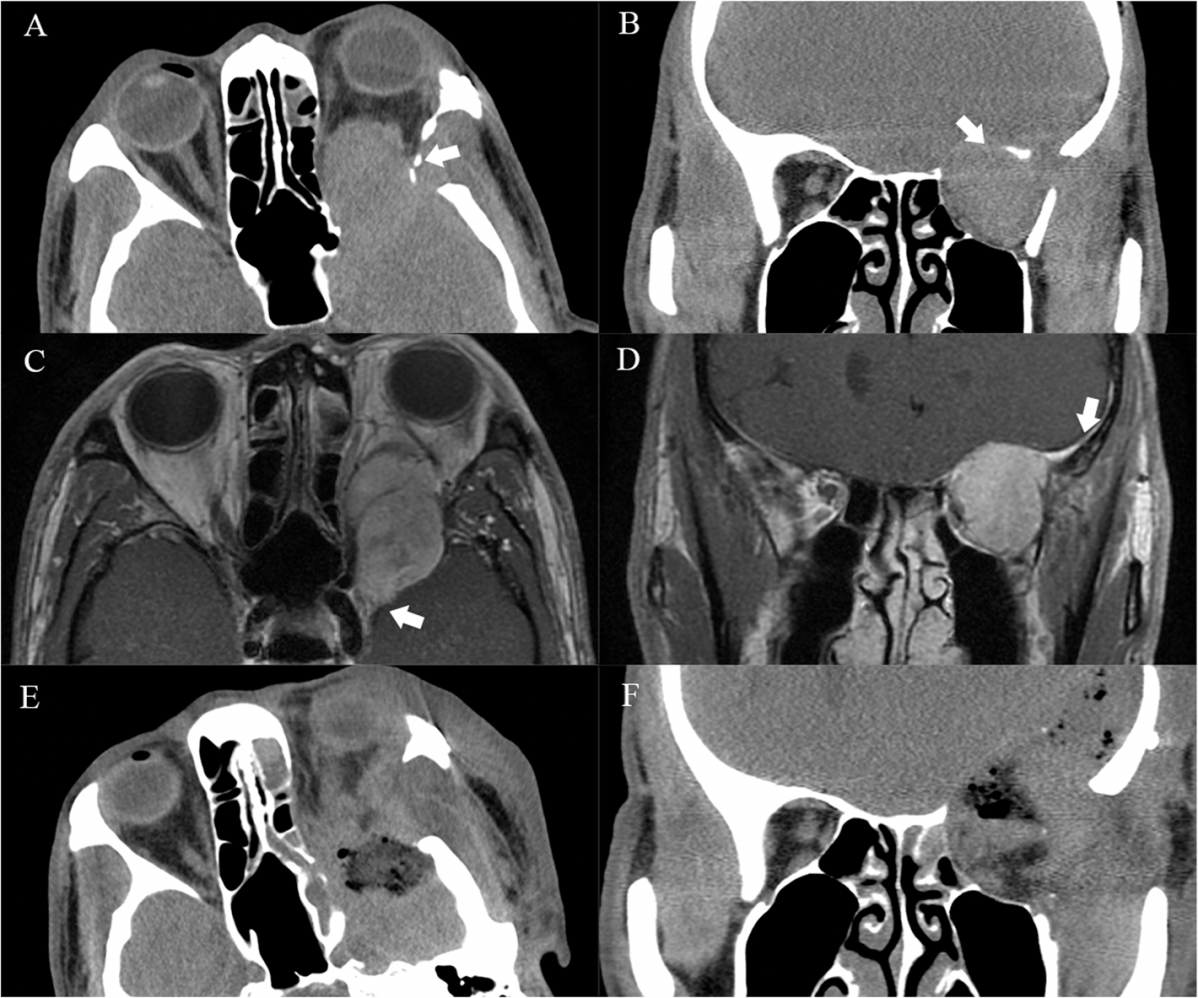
Case 6. The patient was a 48-year-old with a chronic disease process and left exophthalmos for more than 10 months. The patient underwent an operation (Transfronto-orbital approach) 6 years ago. a, b: CT showed that the tumor involved the bone of supraorbital bone with bone destruction (arrow). c, d: MRI showed that the main body of the tumor invaded the intracranial dura and cavernous sinus through the destruction of the skull base bone (arrow). e, f: After the Transfronto-orbital approach was given to the craniotomy, the MRI re-examinations immediately after the operation showed that the tumors were removed completely. There was no recurrence after 4 years of MRI follow-up
